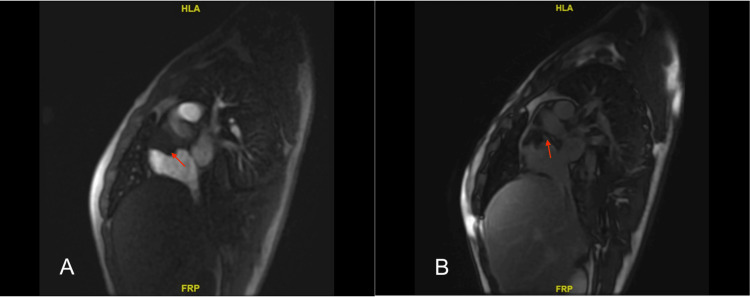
Contrast-enhanced cardiac MRI. A: Solid mass in the right atrium (red arrow); B: Late heterogeneous enhancement of mass, suggesting thrombus is less likely (red arrow)
